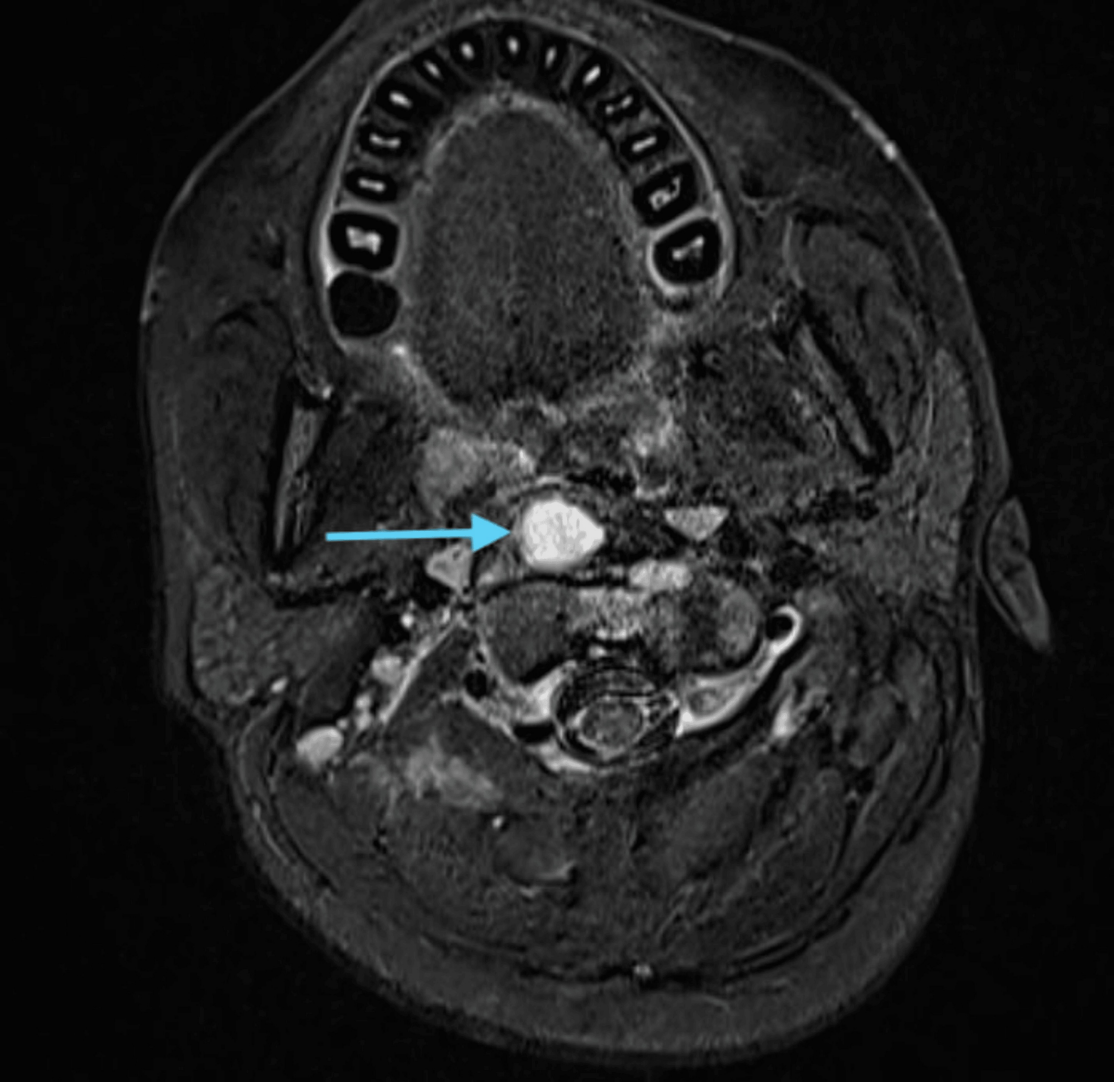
MRI cranio-vertebral junction (contrast) - axial plane A heterogeneously soft tissue component measuring approximately 20 x 36 x 2 mm (AP x TR x CC) (blue arrow) is noted in relation to the foramen magnum on the right side and the right atlanto-occipital joint, right lateral atlanto-axial joint with osteomyelitis affecting clivus, and central non-enhancing hypointense areas of liquefaction or necrosis.
